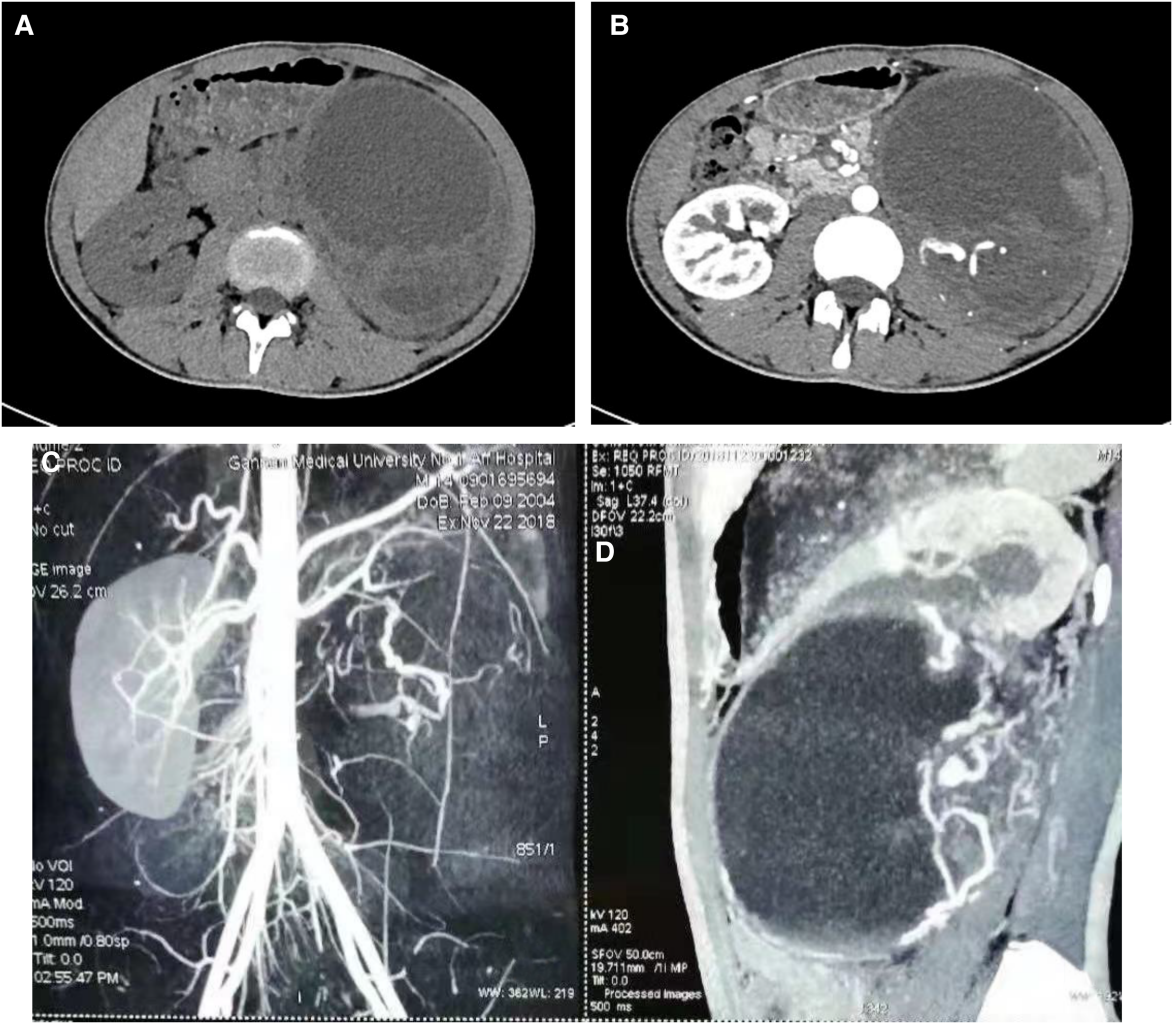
Ct scan of the urinary tract. CT scan of the venous phase of the left renal tumor (A), enhanced CT scan of the arterial phase of the left renal tumor (B), angiography of a tumor in the left kidney (C). Sagittal view of the mass (D).
